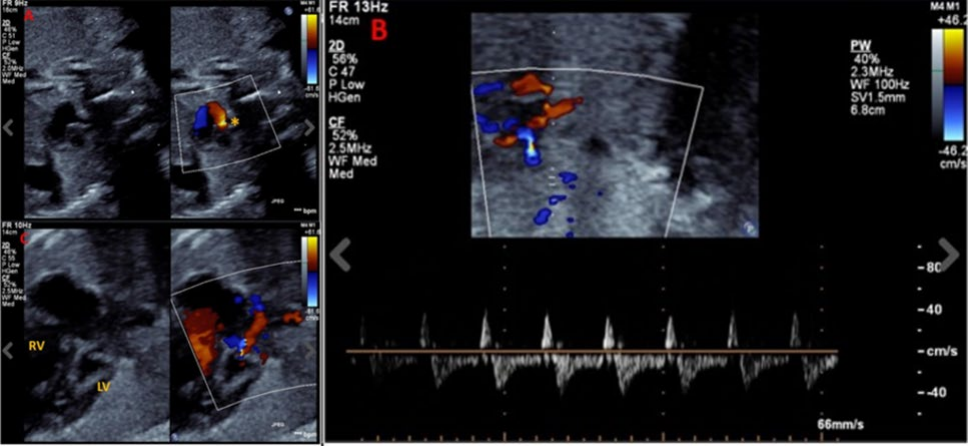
Fetal echocardiogram images at 30 weeks gestation: A- a small, restrictive superior atrial communication was seen with left-to-right shunting (asterisk), B- pulmonary vein Doppler showing significant reversal, with a forward: reverse VTI ratio of 3:1 and C- color compare image showing severely hypoplastic left ventricle and mitral valve. There was significant mitral regurgitation. *RV* right ventricle, *LV* left ventricle
